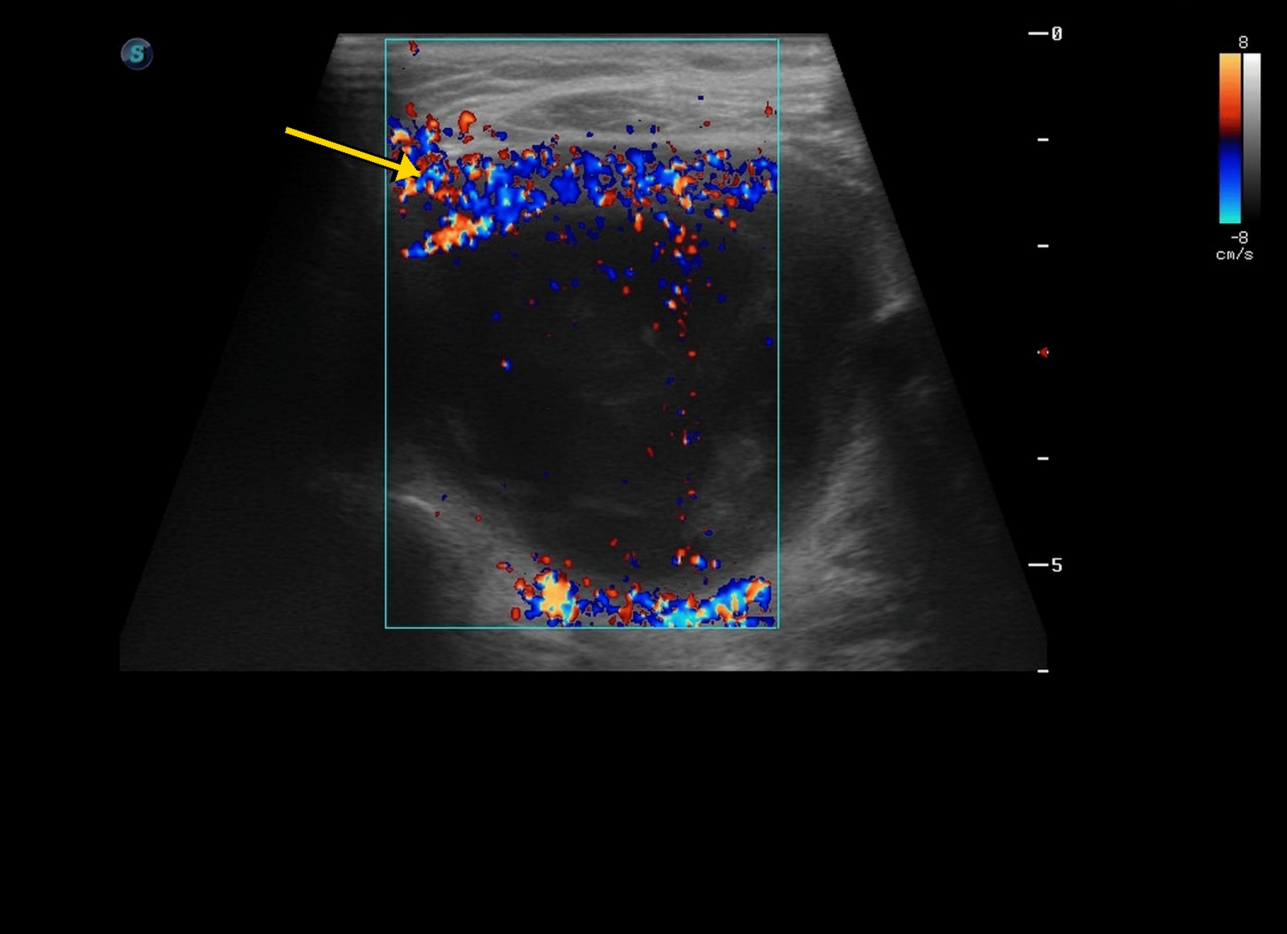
Color Doppler ultrasound of the re-expanded lesion. Representative Doppler image demonstrating predominantly peripheral vascularity along the lesion margin without marked internal hypervascularity. The arrow indicates the predominantly peripheral vascularity. This pattern is nonspecific and does not exclude infection, but it may be compatible with a re-expanded or partially organized cystic collection rather than a solid hypervascular inflammatory mass.
